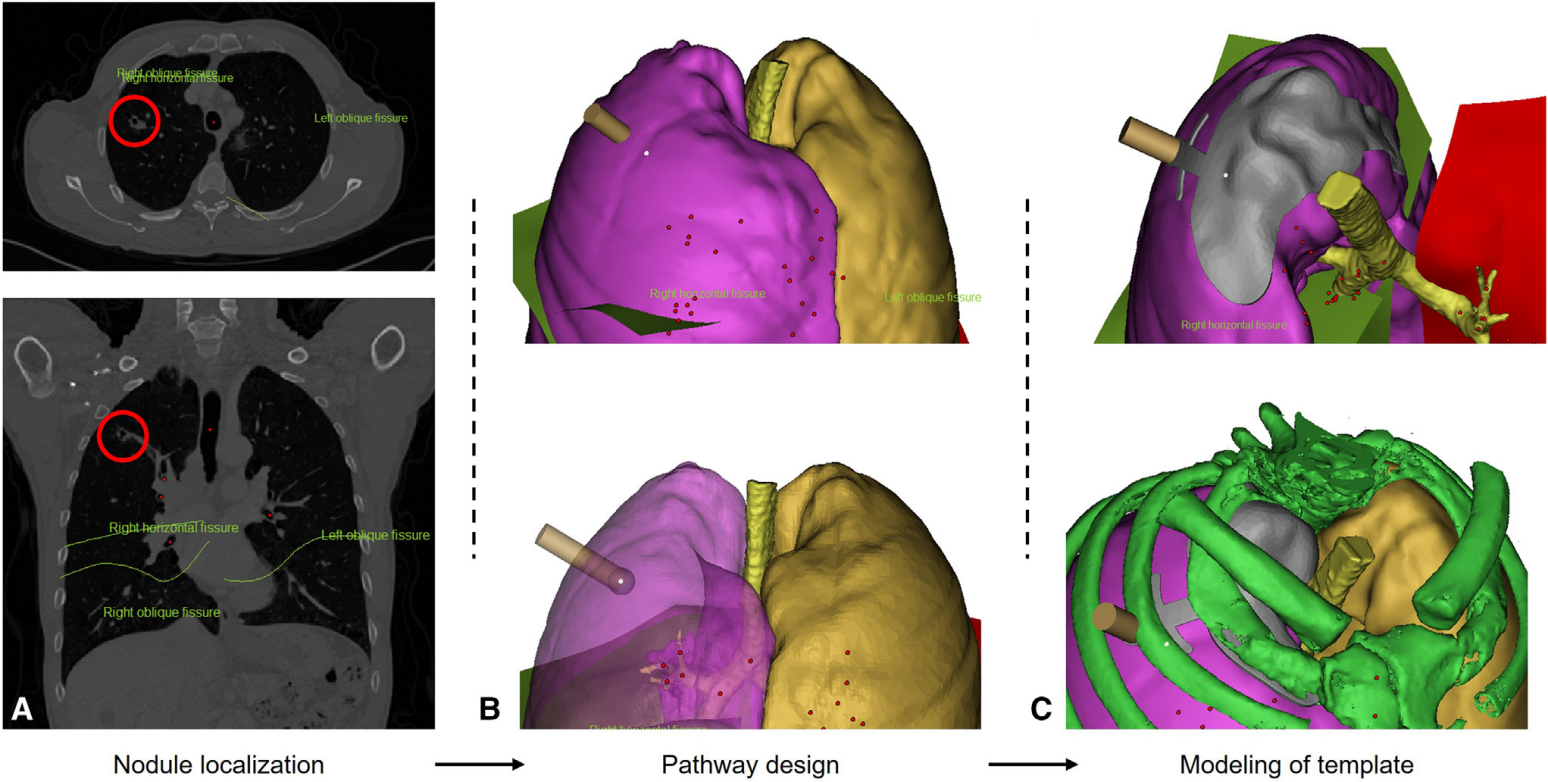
The scheme of template design. A, CT scan showing the location of the nodule in the patient (the *red circle* signified as the target nodule). B, Determining the projection of the nodule on the lung surface based on its location on the 3D modeling. C, Digital modeling of the navigational template.
